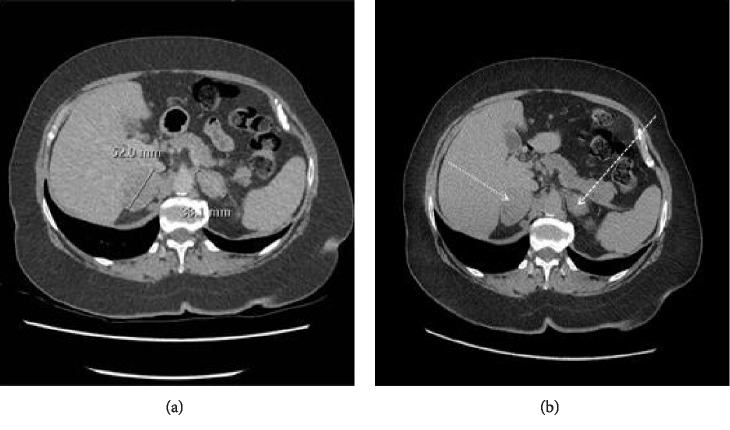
Initial pre- and postcontrast CT image (axial) demonstrating bilateral adrenal metastases (arrows). Nonenhanced CT imaging is actually very helpful because it can dismiss the lesions as benign adenomas.
